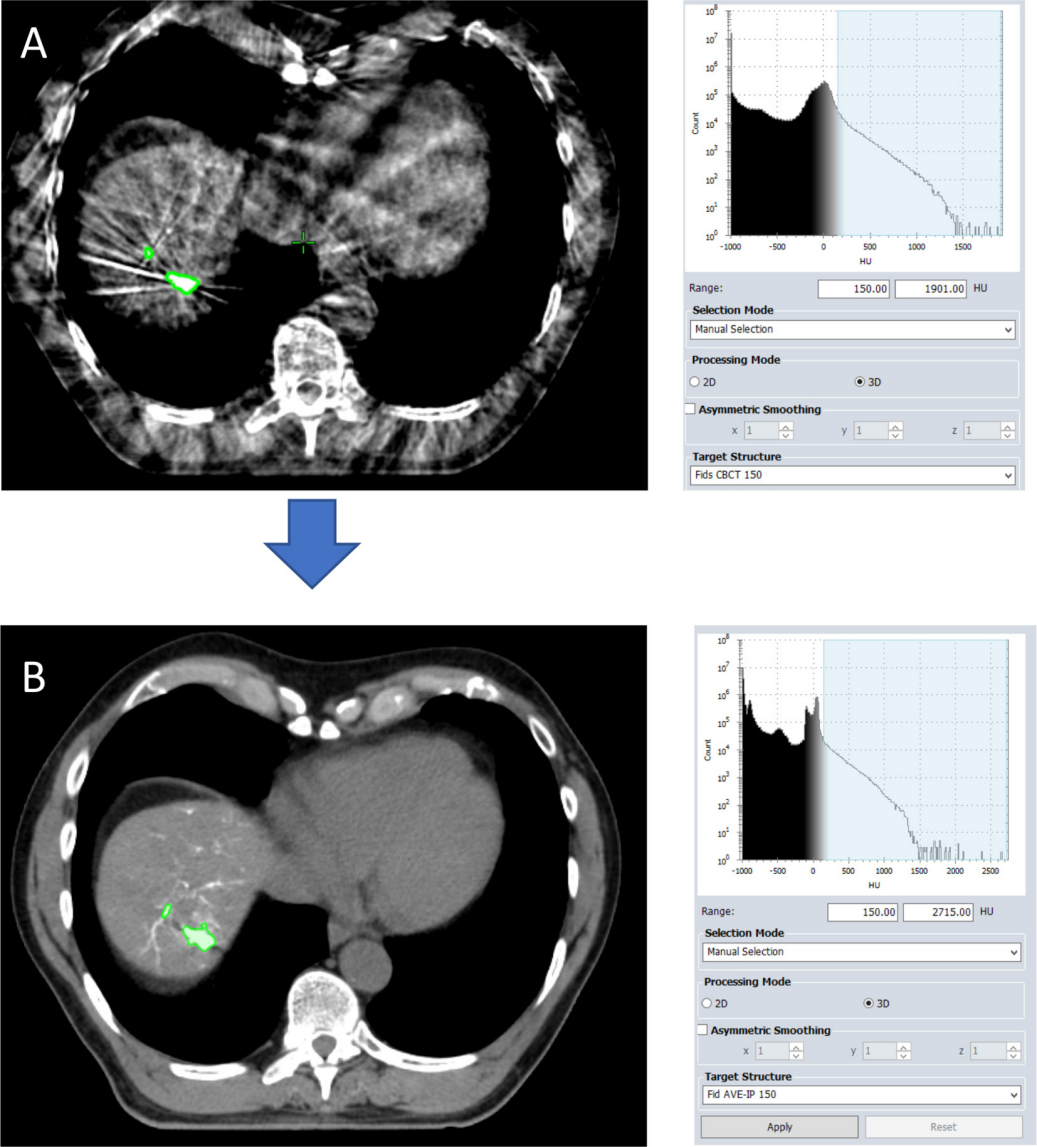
Automated threshold contouring on the CBCT and AVE-IP scans. Areas of beads with a denisty above 150 HU are automatically contoured on the cone-beam CT (CBCT) scan (image A) and average-intensity CT (AVE-IP) scans (image B)
